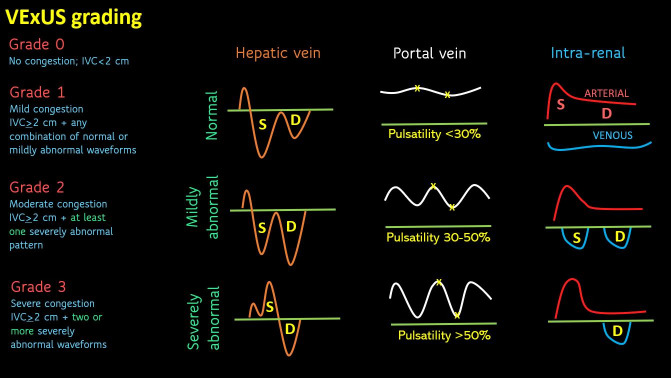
Venous Excess Ultrasound (VExUS) grading system to quantify systemic venous congestion. Briefly, when the inferior vena cava (IVC) diameter exceeds 2.0 cm, congestion is graded by Doppler abnormalities: systolic wave (S)/diastolic wave (D) ratio <1.0 with the S wave below baseline indicates mild hepatic vein congestion, while an S wave above baseline indicates severe congestion. Portal vein pulsatility of 30–50% denotes mild abnormality, while >50% indicates severe congestion (measured over one cardiac cycle). In the intrarenal vein, distinct S and D waves indicate mild congestion, whereas a D-wave-only pattern signifies severe congestion. Intrarenal vein congestion can also be quantified using flow interruptions or the stasis index, where larger flow gaps indicate more severe congestion. Figure reused with permission from NephroPOCUS.com.
